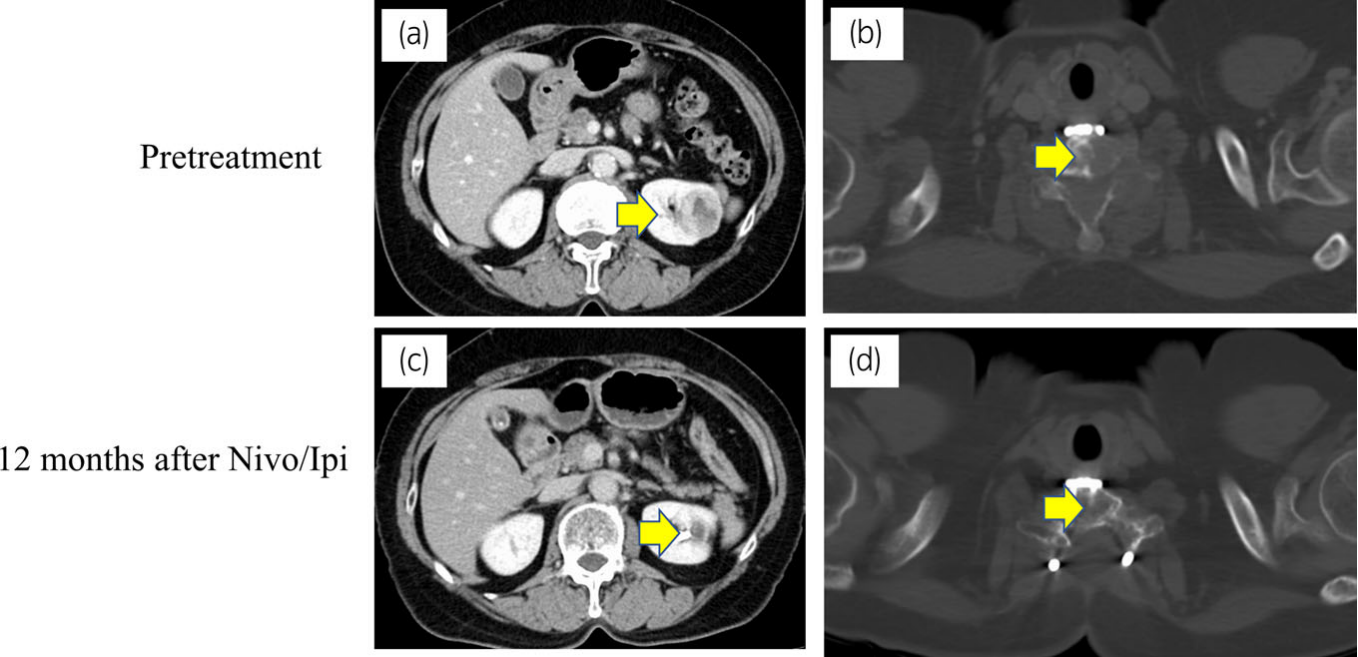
Computed tomography scan findings before treatment and 12 months after nivolumab plus ipilimumab treatment in the primary site (a, c) and cervical bone metastases (b, d).
